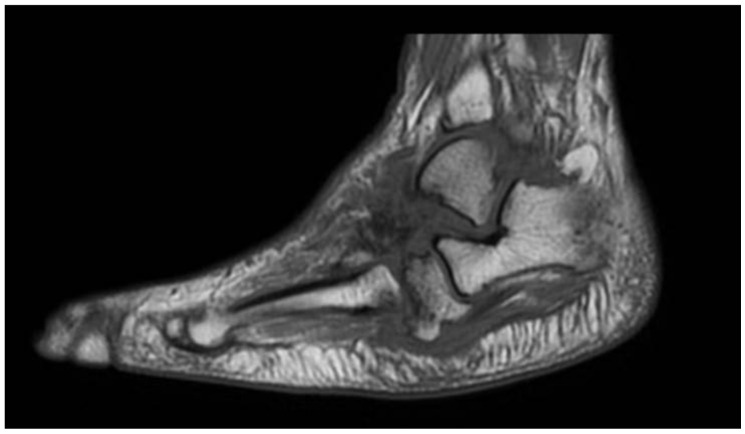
MRI (T1 FSE): Bone marrow edema is characterized by low signal intensity.
